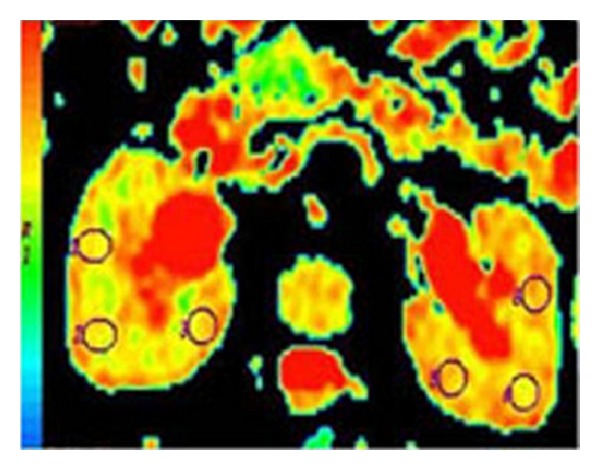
Axial ADC map calculated from echoplanar DWI of hydronephrotic kidneys with a high *b* value. The yellow-green coloration was observed significantly in the right hydronephrotic kidney to be compatible with lower ADC values. The ROIs are placed in three locations: an anterior portion, an intermediate site, and a posterior portion.
